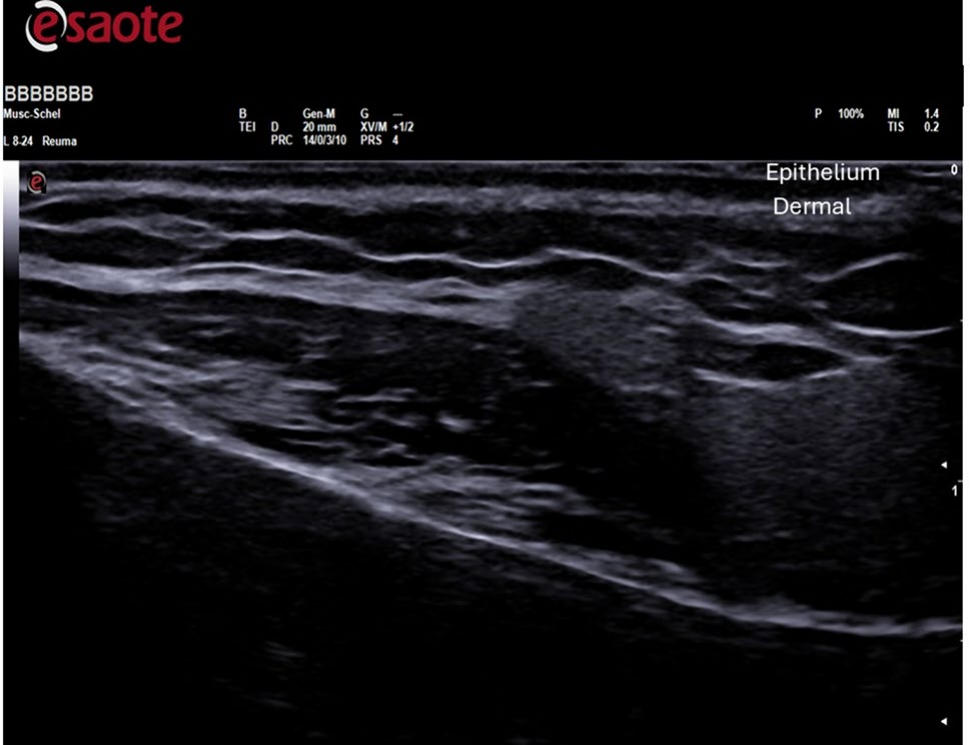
Ultrasound evalutation: the graphs show dermal thickness, epithelium thickness before and after treatment—Ultrasound images after treatment
